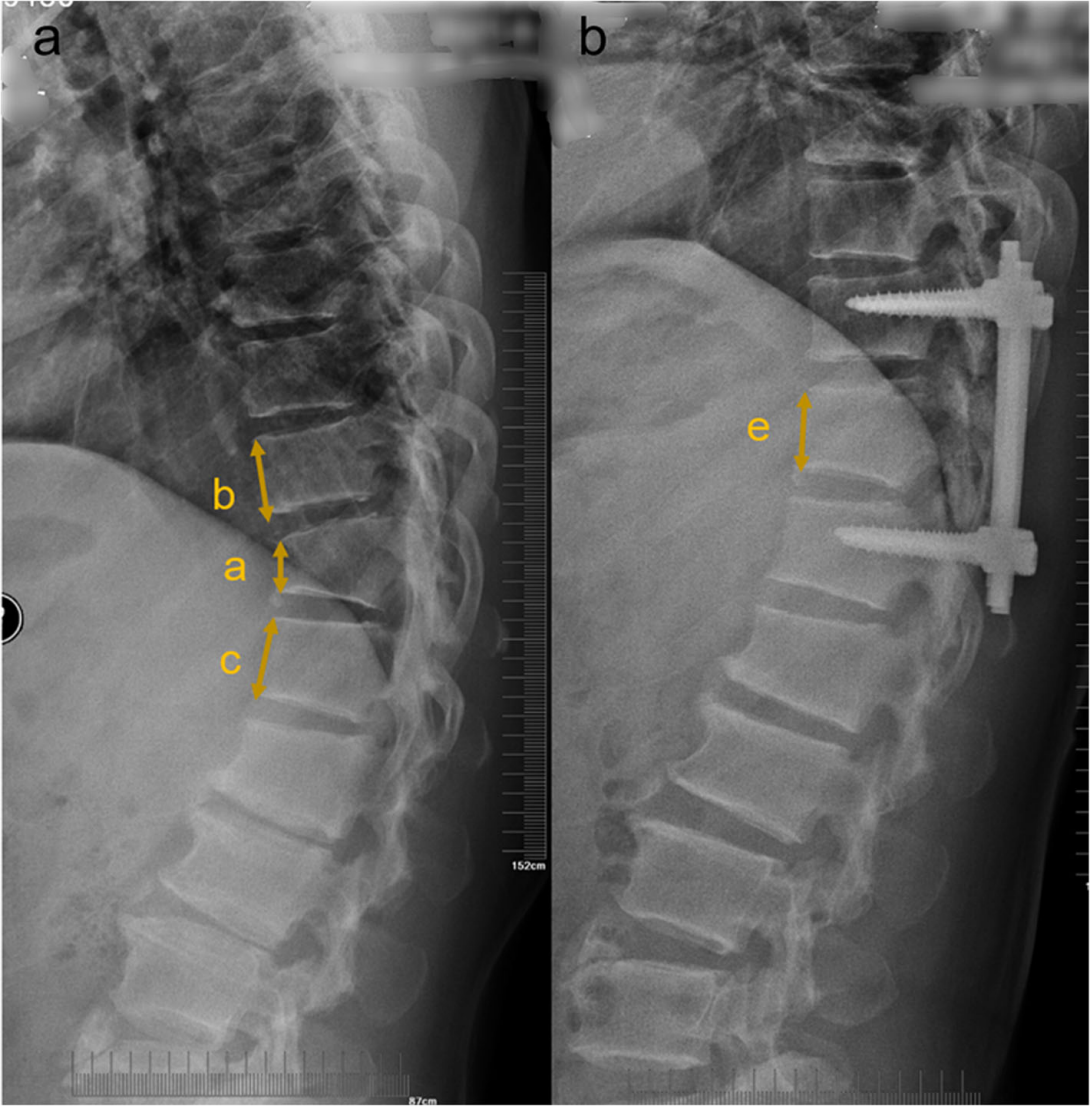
Radiographic measurement of fractured vertebral body. a Preoperative lateral radiograph; *a* is the height of the fractured vertebra; *b* is the upper anterior vertebral height adjacent to the fractured vertebra, and *c* is the lower anterior vertebral height adjacent to the fractured vertebra. b Postoperative lateral radiograph; *e* is the postoperative vertebral body height. *Note*: *d* is the predicted height of each fractured vertebra which is calculated according to the average height of the two adjacent vertebrae (*b* and *c*)
